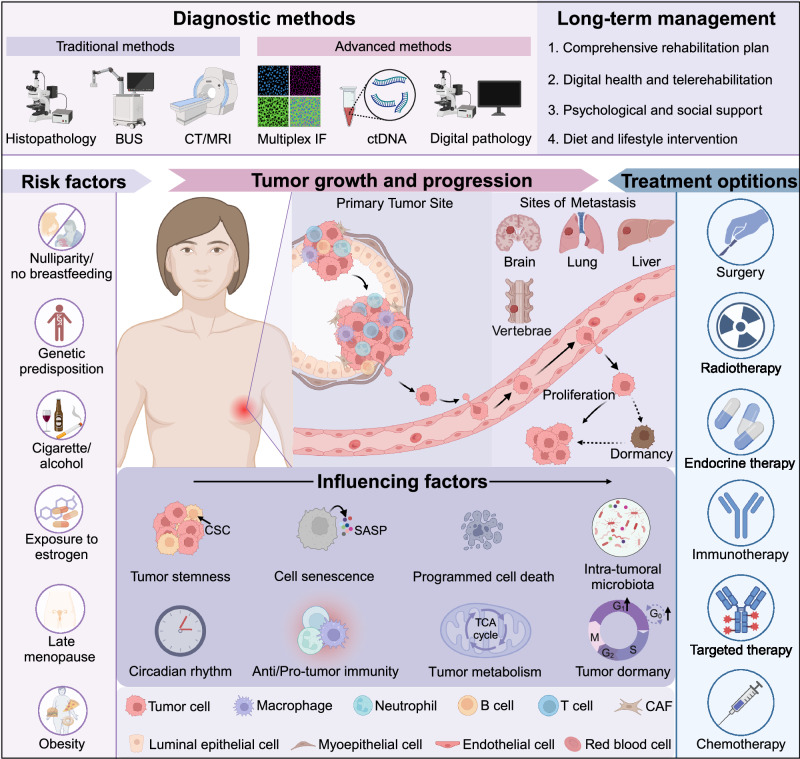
Comprehensive overview of breast cancer pathogenesis and treatment. Breast cancer is one of the most prevalent tumors in women, and its occurrence is associated with a multitude of factors, such as genetic mutations, late menopause, and obesity. The progression of breast cancer is shaped by numerous factors, encompassing both tumor cell characteristics and elements within the tumor microenvironment, whether cellular or non-cellular. In recent years, there have been significant advancements in diagnostic technologies for breast cancer. Alongside traditional imaging techniques and pathological diagnosis methods, liquid biopsy, and multiple immunofluorescence assays, digital pathology approaches are gradually being incorporated into clinical practice. Treatment options for breast cancer are diverse, and recent clinical studies emphasize the importance of individualized and precision treatments. Long-term follow-up management of breast cancer patients is also crucial, as it may impact both the therapeutic outcomes and enhancing patients’ quality of life. BUS B-scan ultrasonography, CT computed tomography, MRI magnetic resonance imaging, IF immunofluorescence, ctDNA circulating tumor DNA, CSC cancer stem cell, SASP senescence-associated secretory phenotype, TCA cycle tricarboxylic acid cycle. The figure was created with Biorender.com
